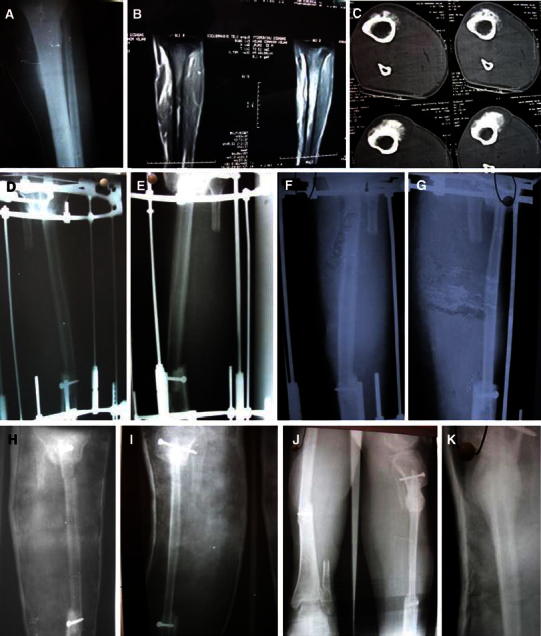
Male 25-year-old patient with bone sarcoma of the tibia: a preoperative plain X-ray; b, c preoperative MRI for diagnosis and staging; d, e postoperative X-ray with Ilizarov external fixator after fixation of the fibula with two interfragmentary screws; f, g plain X-ray taken six months postoperatively; h, i stress fracture after the Ilizarov fixator and above-knee cast was applied; j X-ray showing union of stress fracture; k plain X-ray taken 18 months postoperatively, showing fibula hypertrophy
